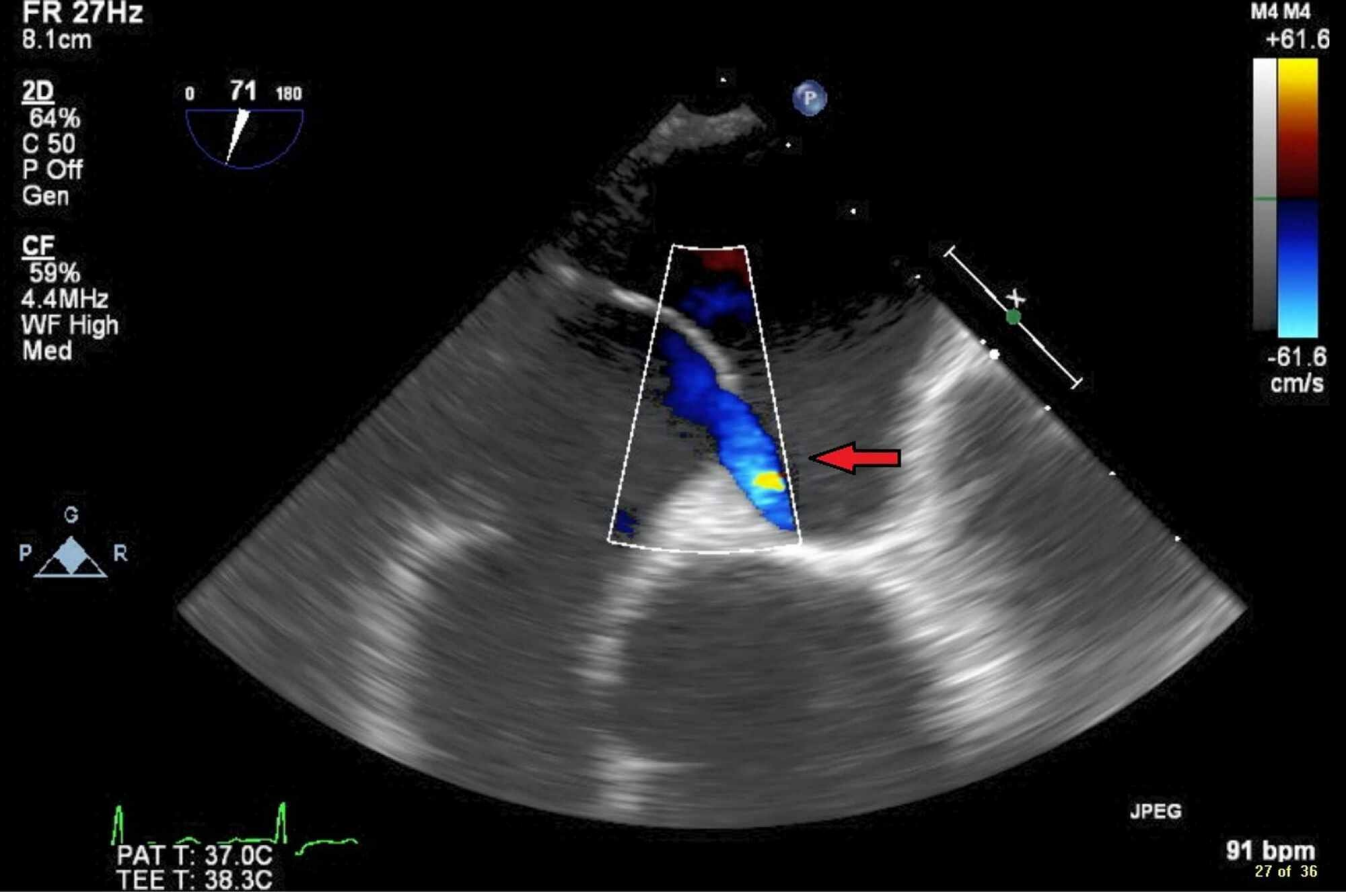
TEE image of PFO demonstrated by color Doppler revealing right-to-left inter-atrial shunting at rest (red arrow). TEE, transesophageal echocardiogram; PFO, patent foramen ovale.
